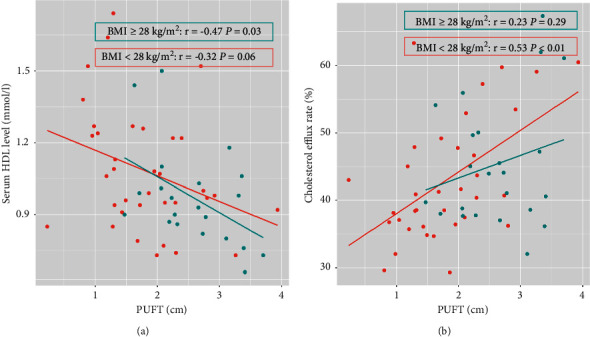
Correlations between para-perirenal ultrasonographic fat thickness (PUFT) and serum high-density lipoprotein (HDL) level (a) and cholesterol efflux capacity (b) in the subgroup divided by body mass index (BMI).
